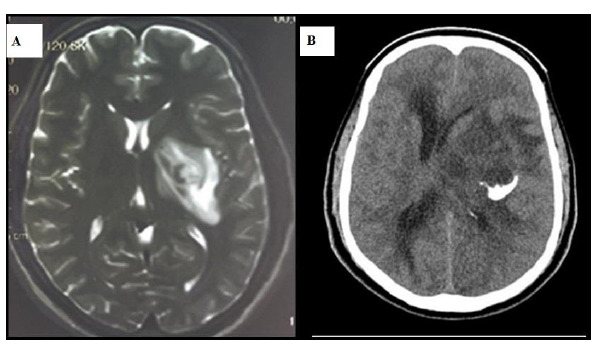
A) Magnetic resonance imaging of the brain with contrast, B) Computed tomography scan of the head without contrast
